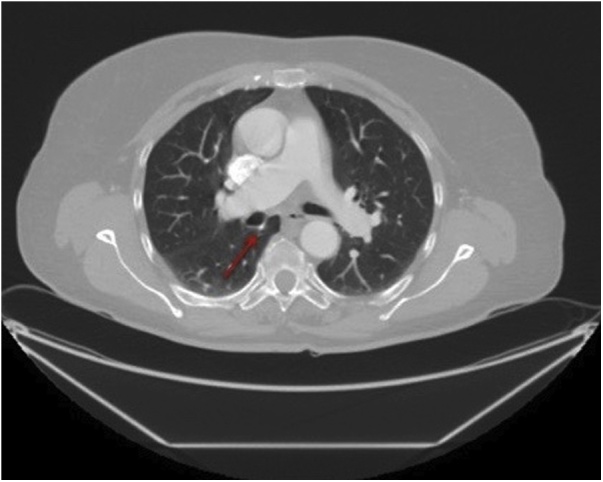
Retrospective review of chest CT shows a venous branch (red arrow) behind the bronchus intermedius.
